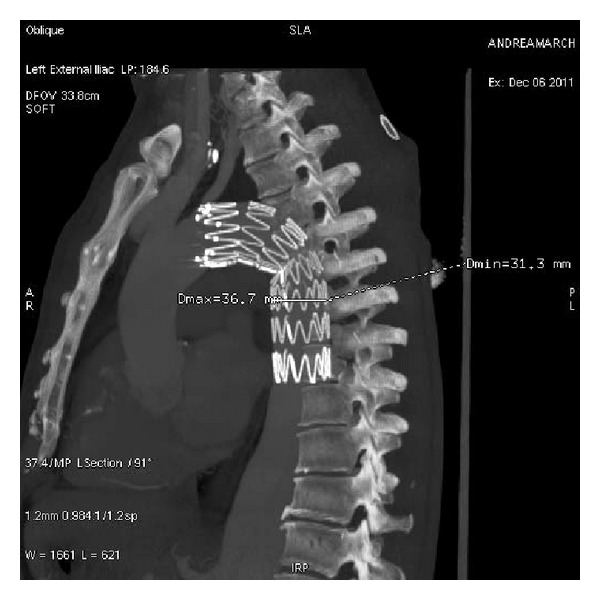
Six-month follow-up CTA reveals that stent-graft placement was intact.
